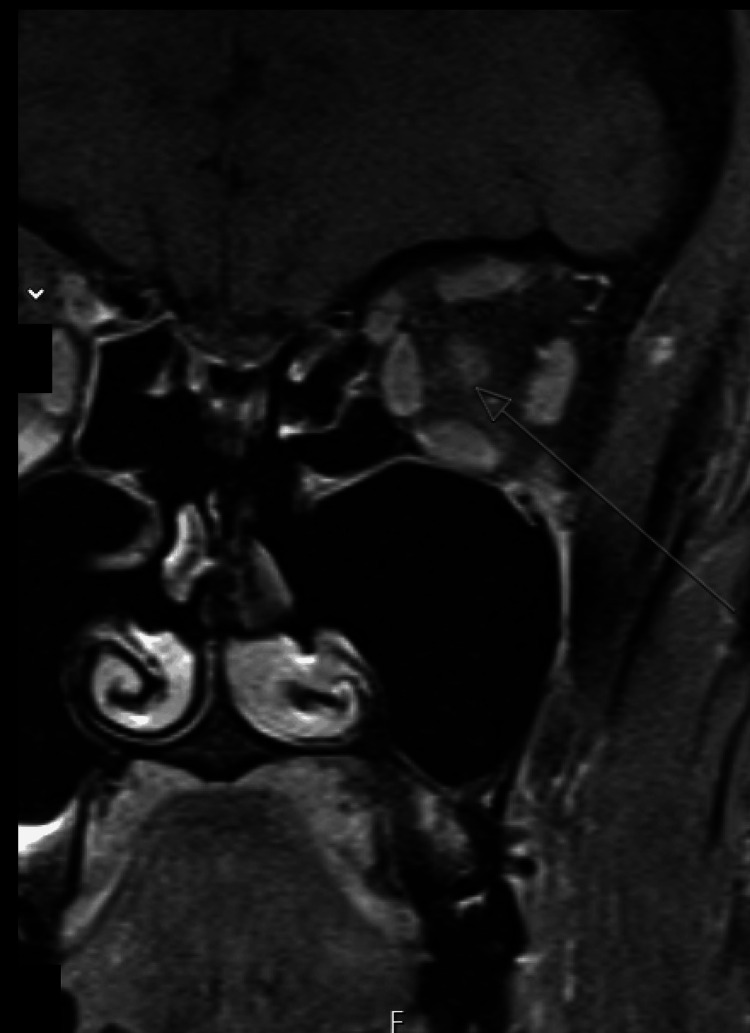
Coronal T1-weighted fat-saturated post-contrast MRI of the orbits demonstrating focal gadolinium enhancement of the orbital segment of the left optic nerve (arrow), consistent with active inflammatory optic neuritis.
